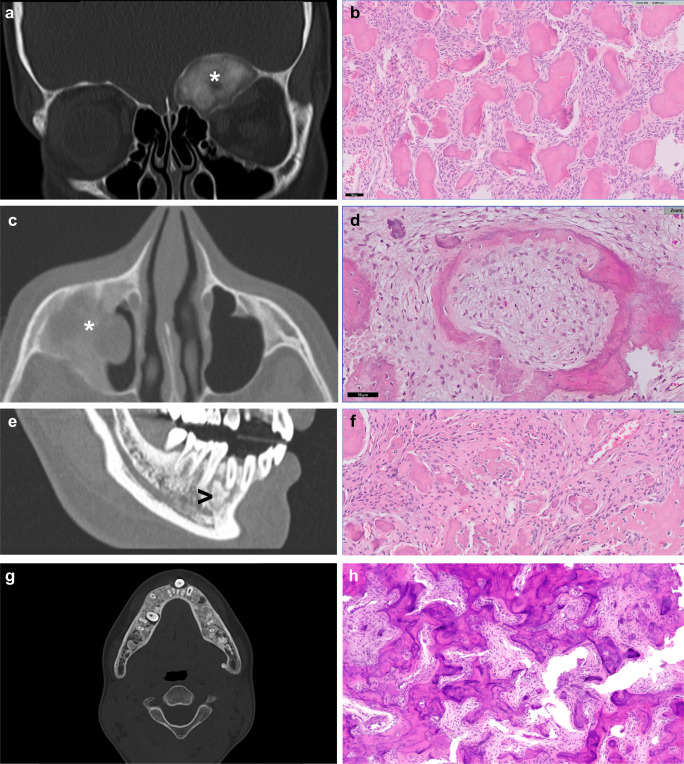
Radiology and morphology examples of fibro-osseous lesions. a Coronal reformatted CT image of a patient with a juvenile psammomatoid ossifying fibroma showing a well-defined ossifying lesion arising from the superior margin of the left orbit (asterisk). The lesion is surrounded by a thin ossified shell. b Morphology of juvenile psammomatoid ossifying fibroma showing a cellular bland spindle cell component and characteristically small spherical ossicles (psammomatoid bodies) of bone rimmed with more flattened osteoblasts. c Axial CT image of fibrous dysplasia located in the right maxillary sinus showing a mass (asterisk) with typical ground-glass appearance without cortical interruption or soft-tissue extension. d Typical morphology of fibrous dysplasia with woven bone trabeculae mimicking Chinese script letters and Sharpey’s fibres that radiate into the surrounding cellular stroma. e Reformatted CT image of a (focal) cemento-osseous dysplasia showing the ossifying lesion near the root of right first premolar in the mandible (arrowhead). f Morphology of a cemento-osseous dysplasia showing a fibroblastic stroma with variable cellularity and a heterogeneous osseous component composed of woven bone and more cementum-like material. g CT scan of a patient with familial gigantiform cementoma showing bilateral massive involvement. h Familial gigantiform cementoma showing irregular trabeculae of woven bone and monomorphic spindle cells
